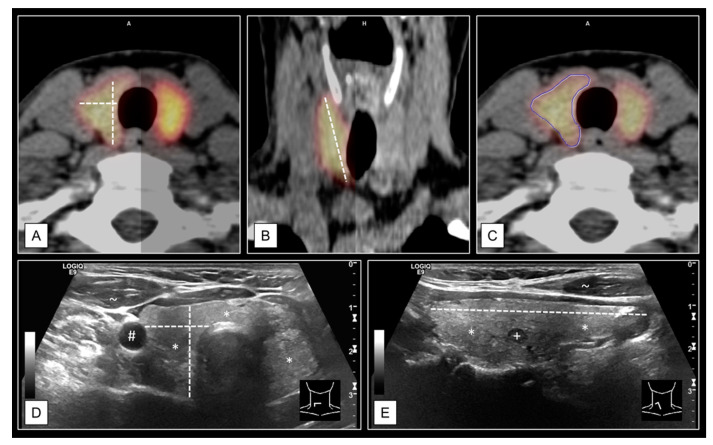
Thyroid gland volume determinations on computer tomography (CT) and ultrasound (US). The example shows a slightly enlarged right thyroid lobe (11.8 mL) of a 69-year-old female. Measurements were recorded on the axial and coronal planes for ellipsoid model determinations on CT (A,B). The corresponding I-124- positron emission tomography (PET) images were superimposed to the CT images for the purpose of optimal accuracy due to the poor soft-tissue contrast of low-dose CT scans. The CT-to-PET ratio was optimized for each measurement (examples: A, left side 80:20, A, right side 50:50; B, left side 75:25, B, right side 100:0). Furthermore, the contours of both thyroid lobes were manually traced via syngo.via™ software (Version VB40, Siemens Healthcare GmbH, Erlangen, Germany), on every single axial plane of PET/CT images (C). US volume determinations were carried out according to the ellipsoid model method on axial (D) and sagittal (E) plane for each thyroid lobe. Labels: * thyroid tissue, + thyroid nodules (TN), # carotid artery, ~ muscle tissue.
